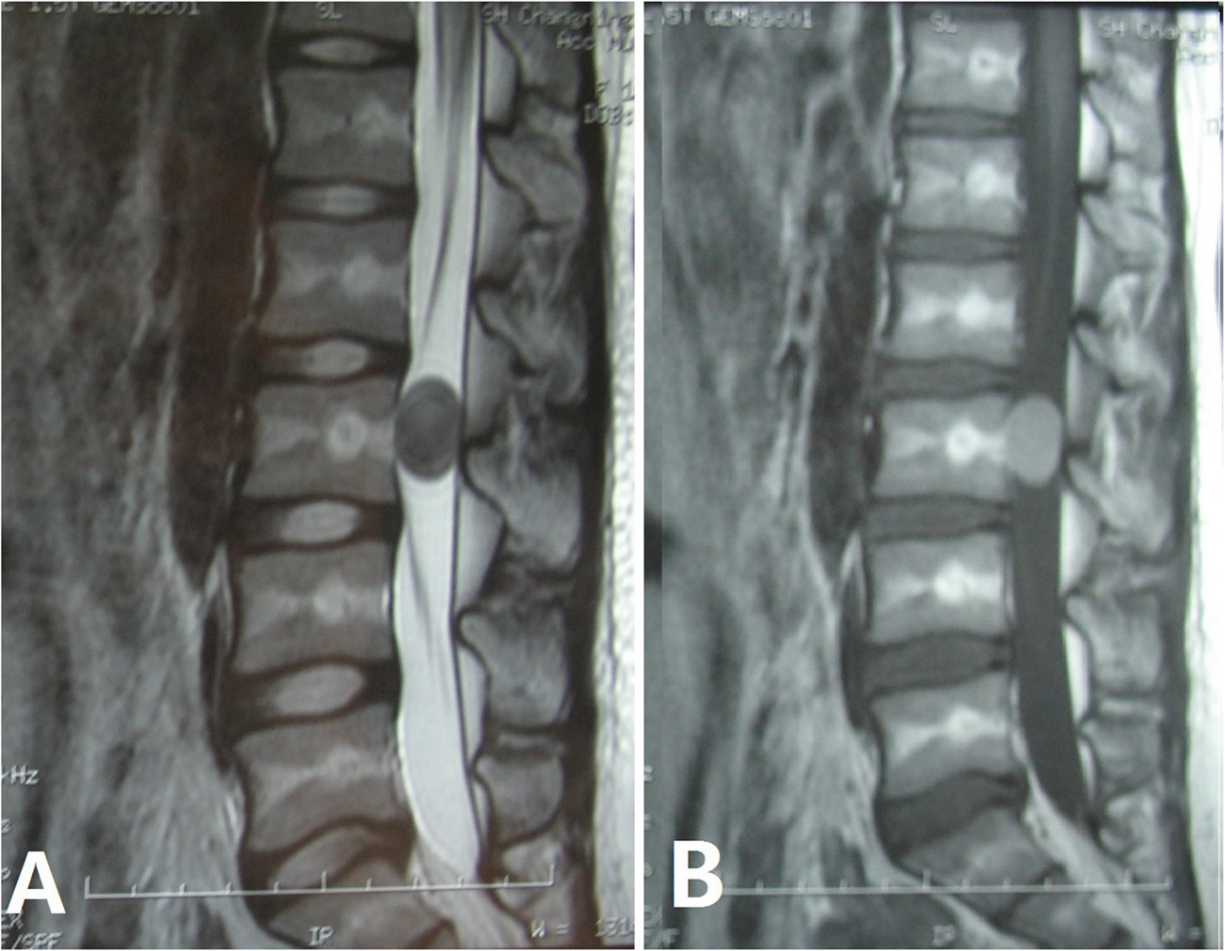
MRI images of an epidermoid cyst with atypical imaging (case 2). (A) T2-weighted image. The epidermoid cyst was hypointense. (B) T1-weighted image. The tumor was hyperintense.
